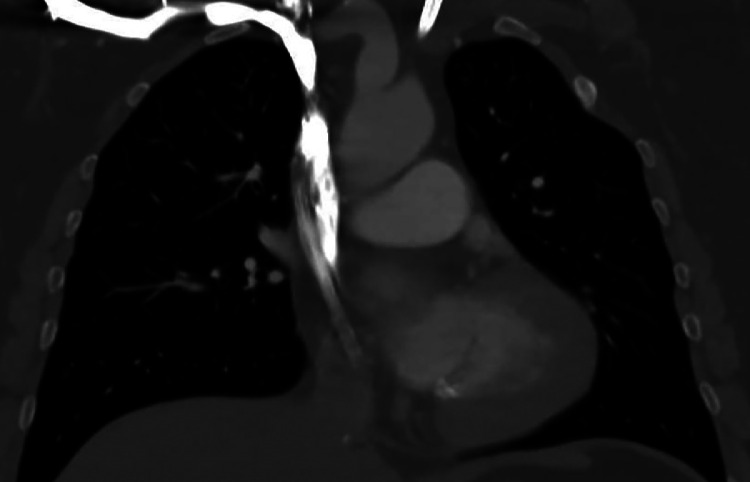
Follow-up 4 months after intervention: no recurrent thrombus in the superior vena cava after mechanical removal of thrombus and dislodged catheter fragments. Coronal contrast-enhanced computed tomography (CT) obtained 4 months after successful mechanical thrombectomy shows restored flow in the superior vena cava (SVC) following removal of thrombus and dislodged catheter fragments. The tunneled hemodialysis catheter via the internal jugular vein (IJV) remains in place.
